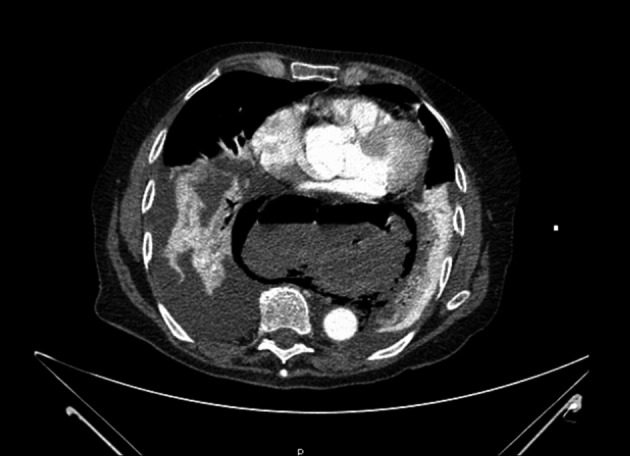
CT images taken after admission. The large hernia within the mediastinum is visible with surrounding air.
